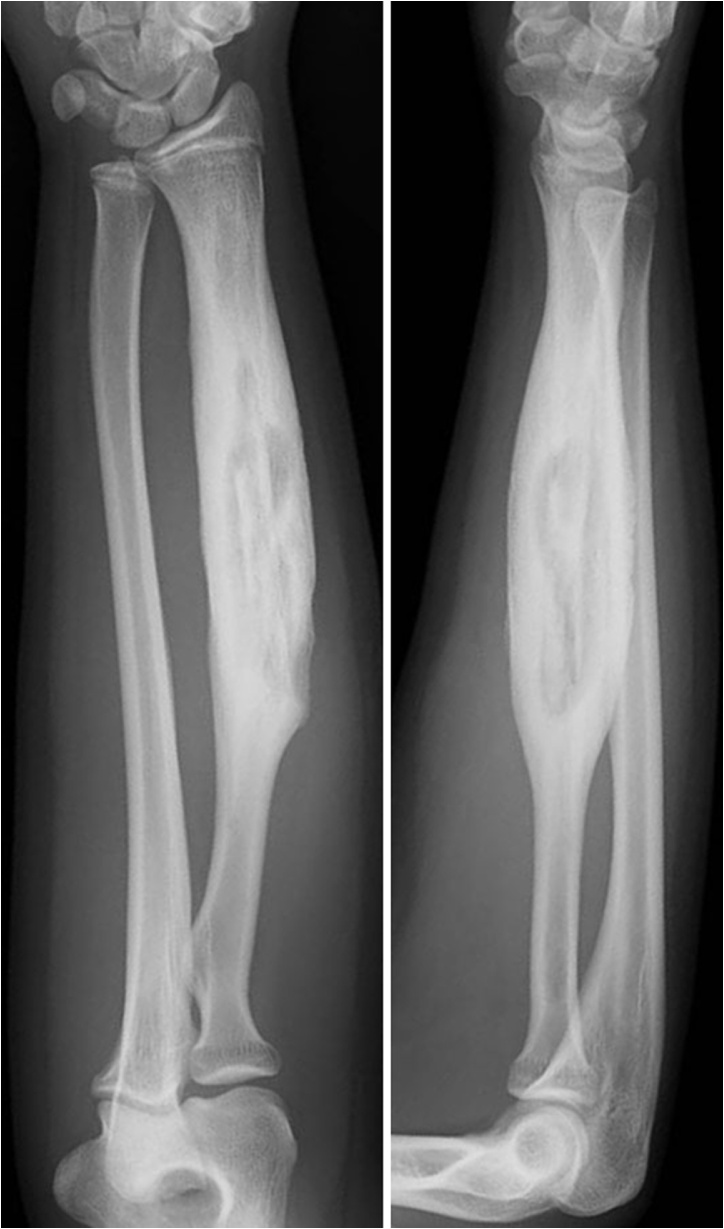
Plain radiograph. A plain radiograph shows hypertrophic cortical bone and a radiolucent lesion in the diaphysis of the right radius.
